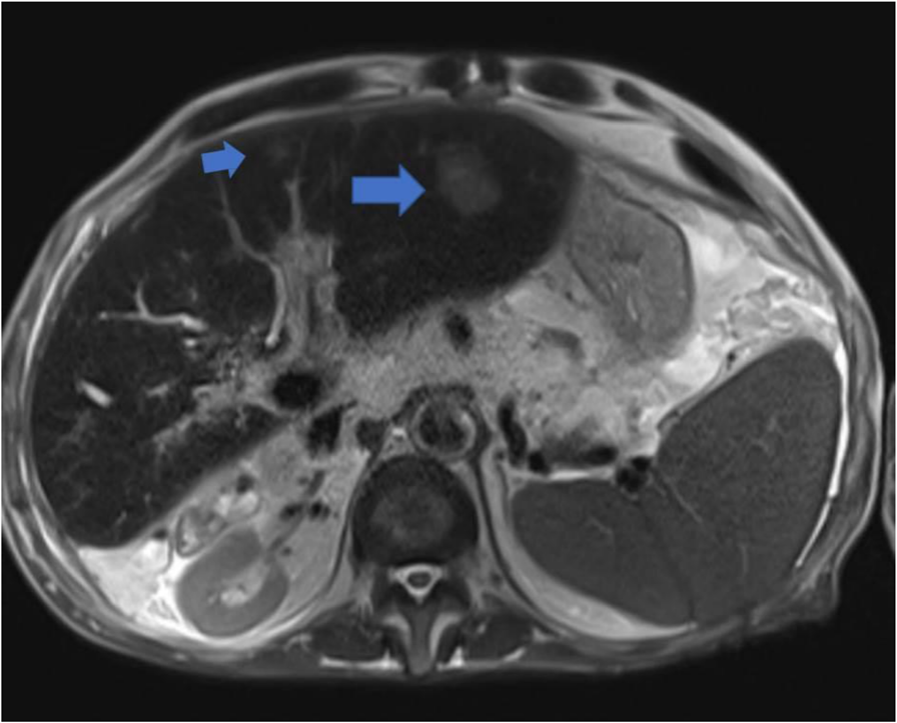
MRI-investigation of the liver 12 months after end of treatment with SVR; a nodule (3 cm diameter) with the characteristic of a HCC was detected in the left liver lobe (arrow), while a further small nodule was suspected (smaller arrow). No signs of cirrhosis were detectable
